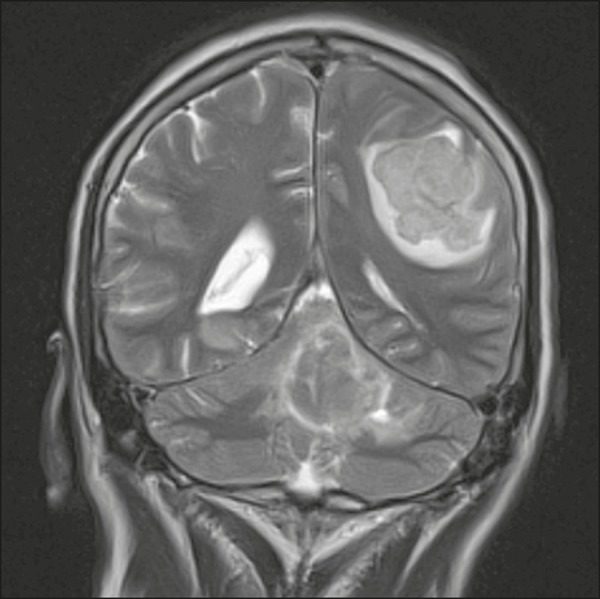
Metastasis. Coronal T2-weighted MRI sequence. Patient with breast
